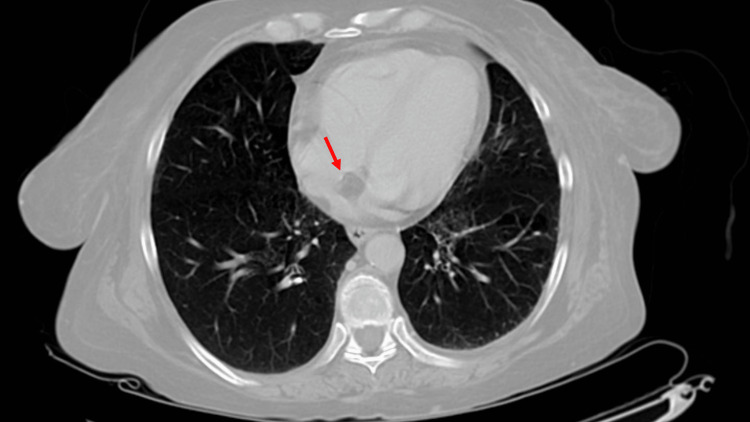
Smooth, non-enhancing, well-marginated 14.9 mm mass visualized on non-contrast computed tomography, characteristically sparing the fossa ovalis and maintaining an elongated shape
